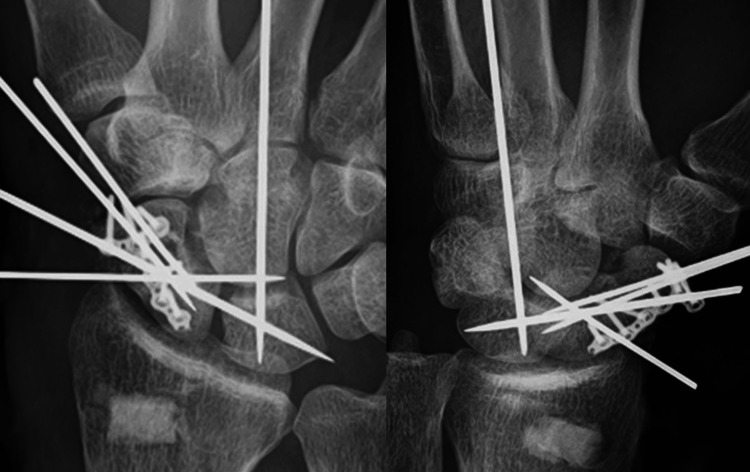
Postoperative radiographs. Postoperative radiographs showing fixation methods and grafting. We harvested autografts from the distal radius and filled the harvested area with artificial bone (beta-tricalcium phosphate).
